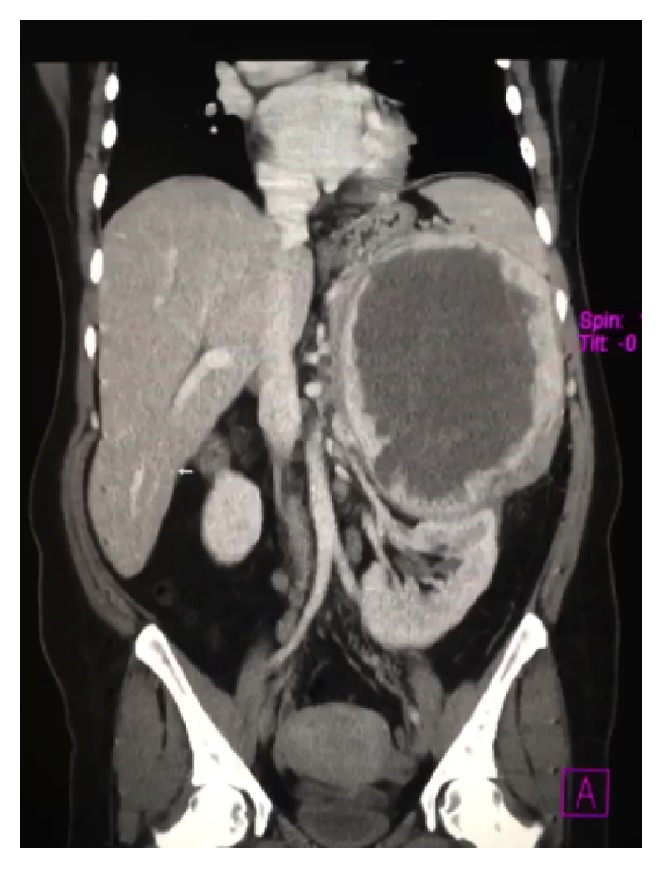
Coronal CT image demonstrating the mass displacing the left kidney inferiorly. Evidence of locally invasive disease was not present.
